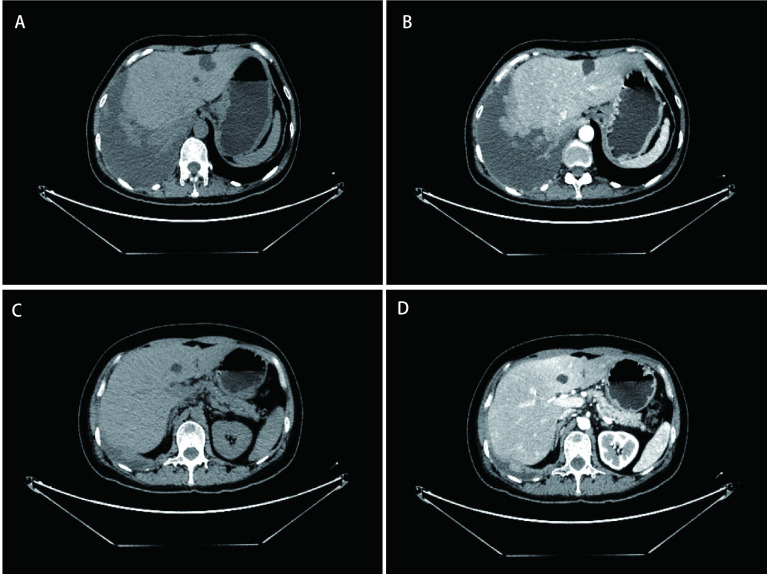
腹部CT平扫+增强示肝内可见多发大小不等低密度影，部分病灶周围似可见稍高强化，不除外转移瘤可能。A、B：腹部CT同一平面平扫与增强对比图像；C、D：腹部CT同一平面平扫与增强对比图像 Plain scan and enhanced scan of abdominal CT showed multiple low-density shadows of different sizes in the liver, and some lesions seemed to have slightly higher enhancement around, excluding the possibility of metastasis. A and B are plain and contrast enhanced images of the same plane of abdominal CT; C and D are plain and contrast enhanced images of the same plane of abdominal CT
